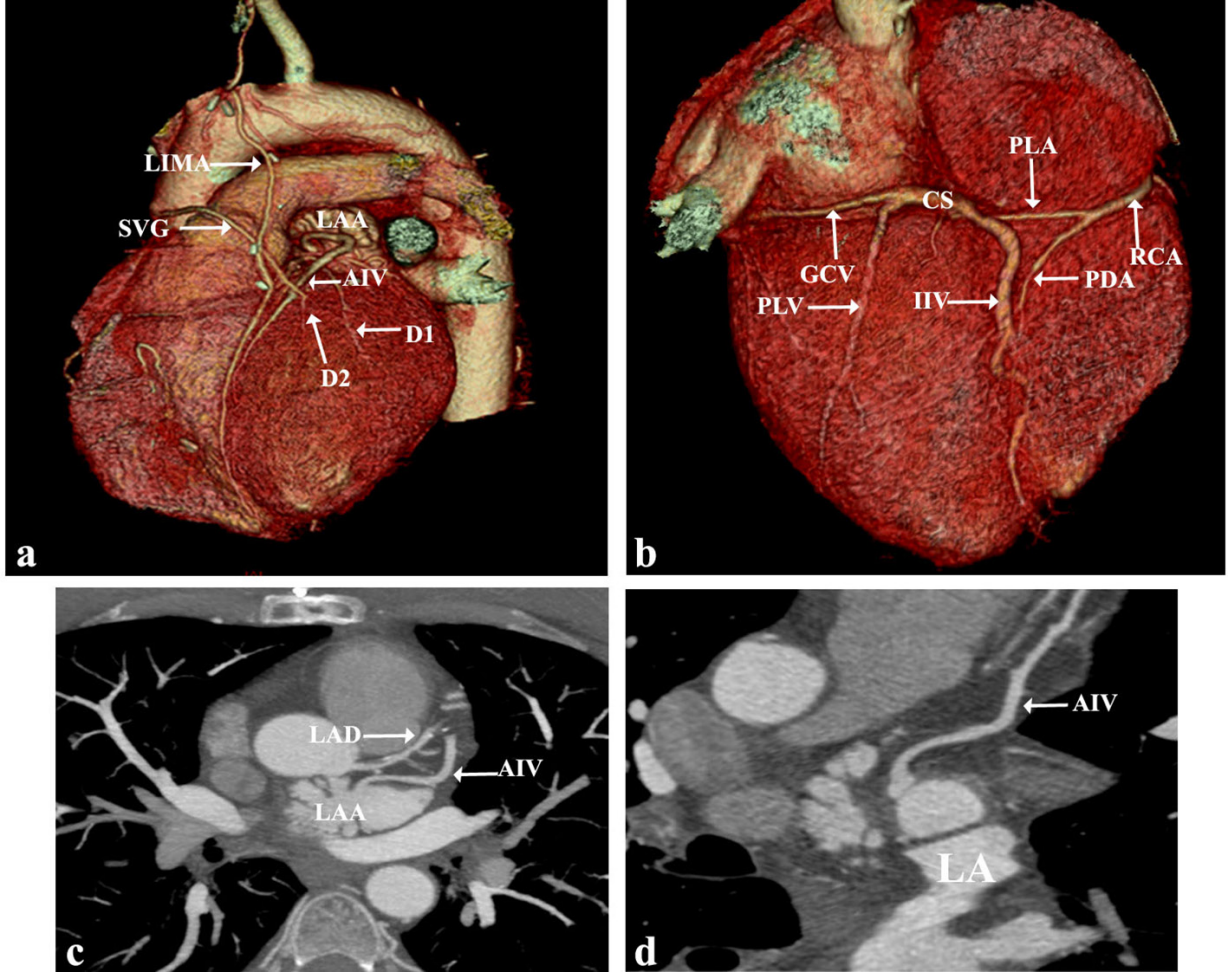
Multidetector coronary CT of a status post CABG: three-dimensional volume rendering images (a, b). Oblique MIP image (c). Curved reformatted image (d). AIV passes parallel to LAD in the anterior interventricular groove and superficial to the first diagonal artery into the left atrium. AIV is connected to GCV by a small venous tributary, and then the GCV is drained normally to CS. GCV: great cardiac vein; CABG: coronary artery bypass graft; AIV: anterior interventricular vein; CABG: coronaryartery bypass graft; GCV: great cardiac vein; IIV: inferior interventricular vein; LAA: left atrial appendage; LAD: left anterior descending artery; MIP: maximum intensity projection.
